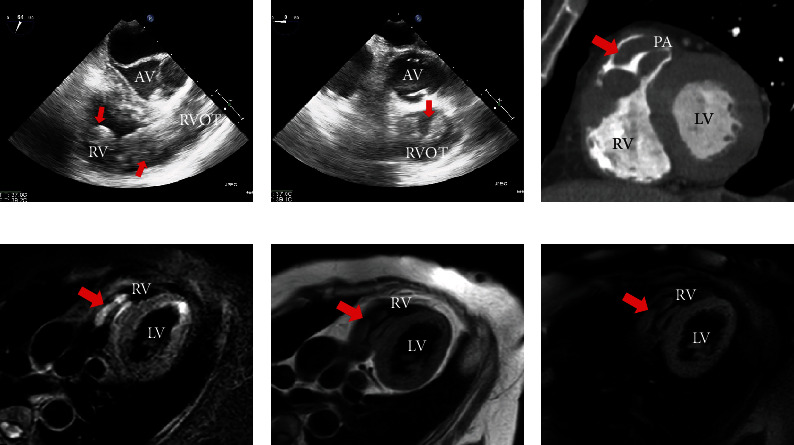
Transesophageal echocardiography, cardiac CT, and MRI appearance. Transesophageal echocardiography showed elongated mass-like structure (arrow) in the right ventricle (a, b). Short axis view of cardiac computed tomography scan revealed a well-defined mass lacking contrast enhancement in the right ventricle below the pulmonary artery valve (c), and cardiac magnetic resonance imaging showed some masses which had hypersignal intensity on fat-saturated T2-weighted image (d), isointensity on T1-weighted image (e), and no suppressed signal on fat-saturated T1-weighted image (f). (AV: atrioventricular; RV: right ventricle; RVOT: right ventricular outflow tract; LV: left ventricle; RV: right ventricle).
